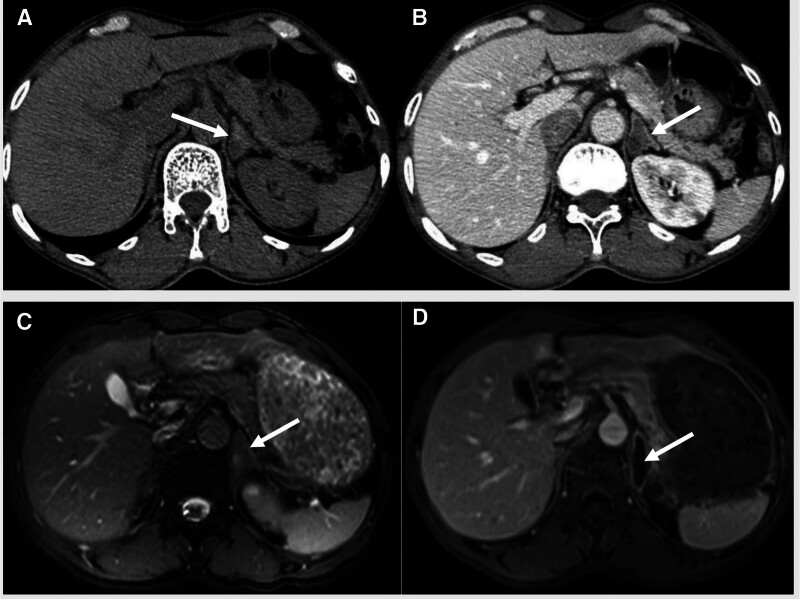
Enhanced computed tomography (CT) and magnetic resonance imaging (MRI) of the patient’s adrenal glands. (A) CT non-enhanced scan; (B) CT enhanced scan; (C) T2WI of MRI; (D) MR enhanced scan. MRI = magnetic resonance imaging.
